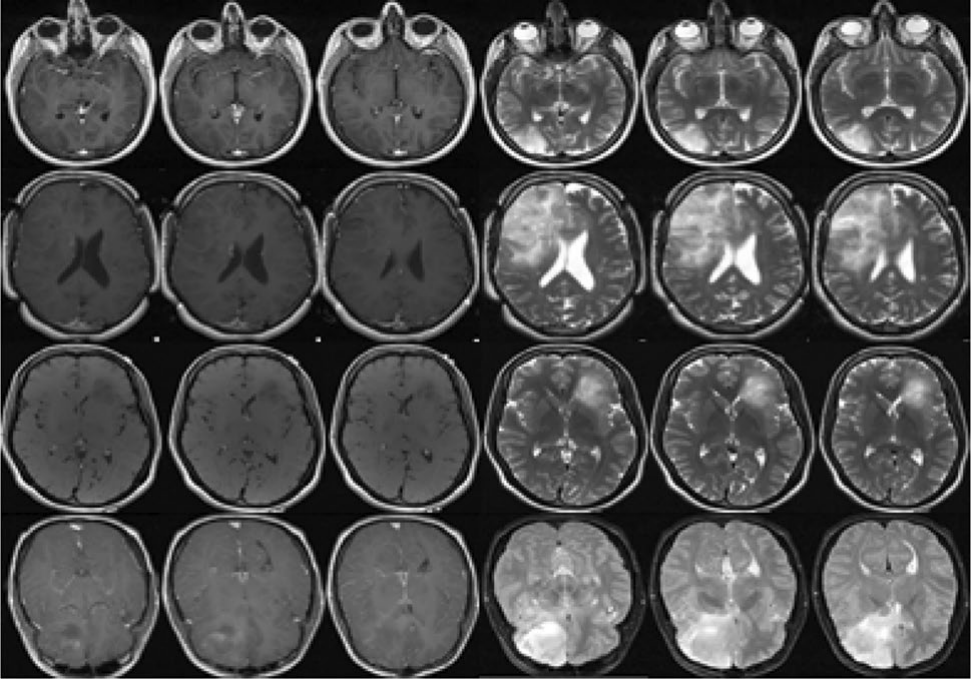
Representative MRI images for four subjects are shown. Column-1 to column-3 are post contrast T1-W MRI; column-4 to column-6 are T2-W MRI. Row-1 and row-2 represent 1p/19q co-deleted G-II and G-III glioma respectively. Row-3 and row-4 represent 1p/19q non-deleted G-II and G-III glioma respectively.
